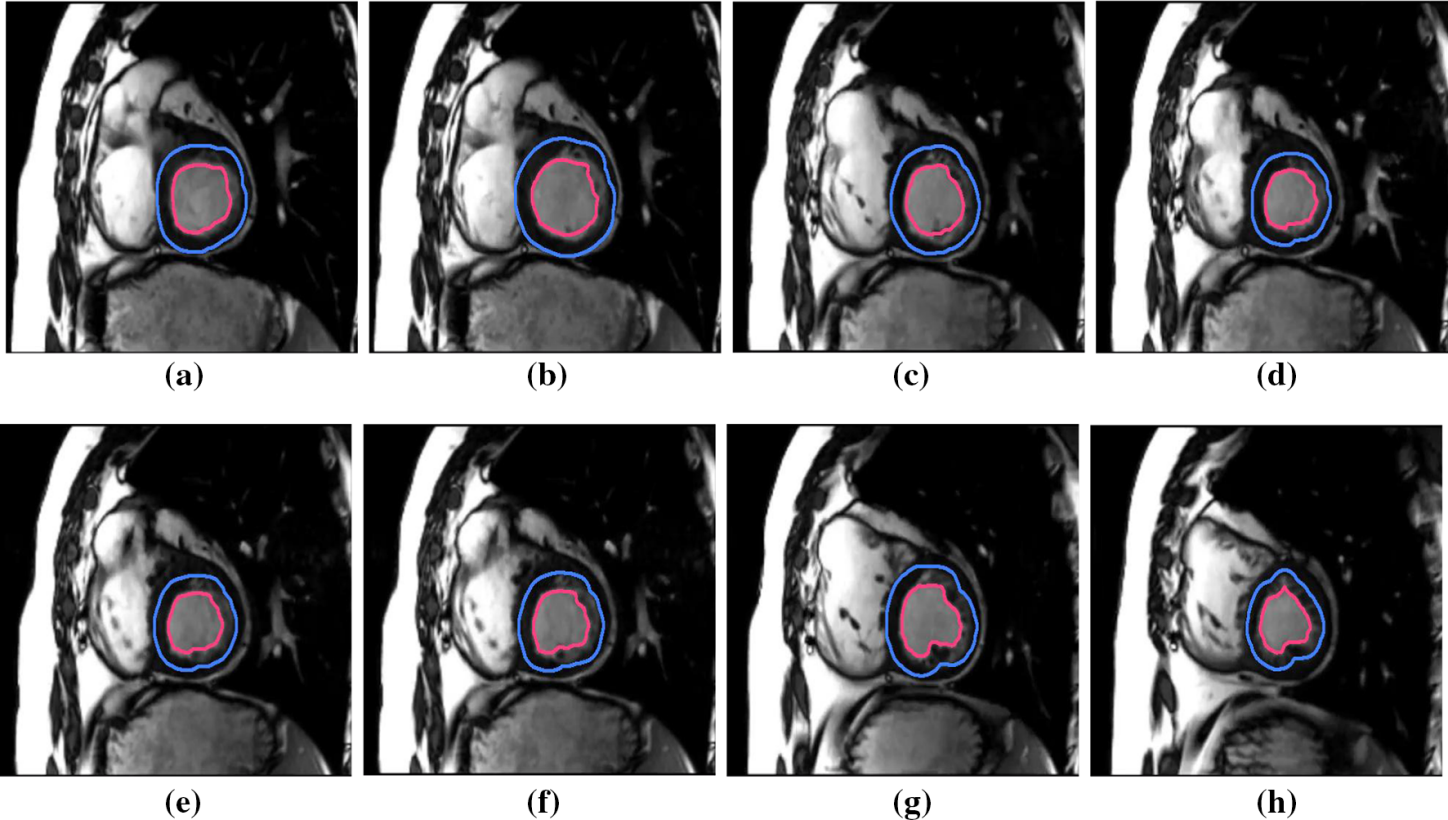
Tracking of left ventricle in high-contrast cine magnetic resonance imaging (high-contrast CMRI) during cardiac surgery
